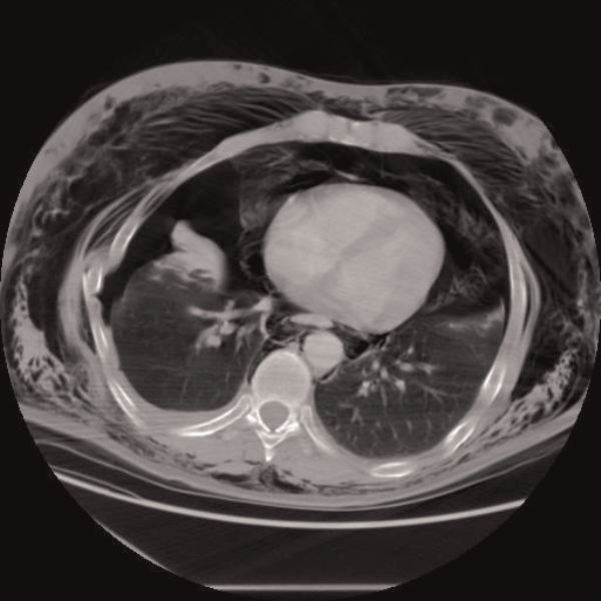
CT showing bilateral pneumothoraces.
